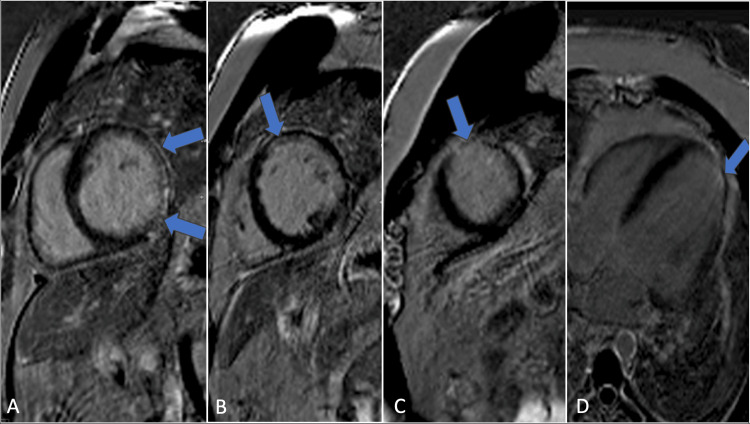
Cardiovascular MRI demonstrated subendocardial delayed enhancement at all levels, including the base (A), mid-cavity (B), anterolateral (C), and apical lateral segments (D). The delayed enhancement was greater than 50% transmurally (blue arrows). Additionally, transmural delayed enhancement was observed in the anterior apical segment (D).
